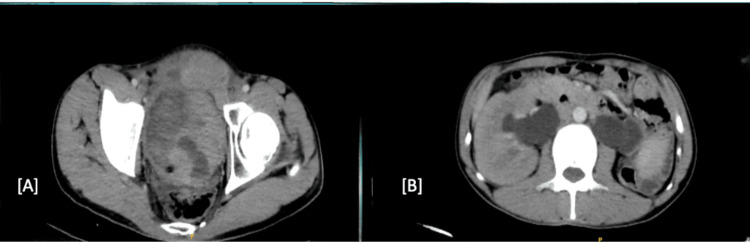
Axial section of contrast-enhanced computed tomography whole abdomen (A) A large heterogeneously enhancing mass lesion in the urinary bladder, which is infiltrating the anterior abdominal wall; (B) Both kidneys show hydroureteronephrosis with a small left kidney.
